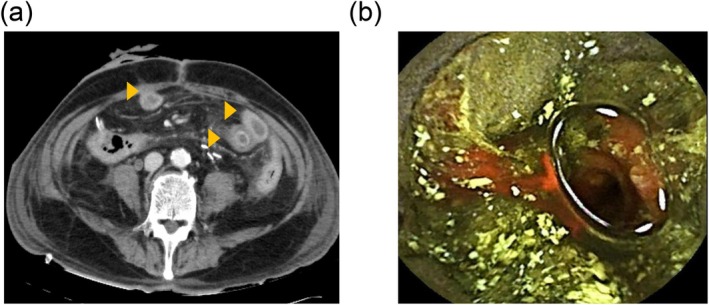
Imaging findings of the small intestine. (a) Contrast‐enhanced abdominal computed tomography showing wall thickening of the small intestine (orange arrows). (b) Capsule endoscopy showing black discoloration of the small intestinal mucosa, suggestive of necrosis.
